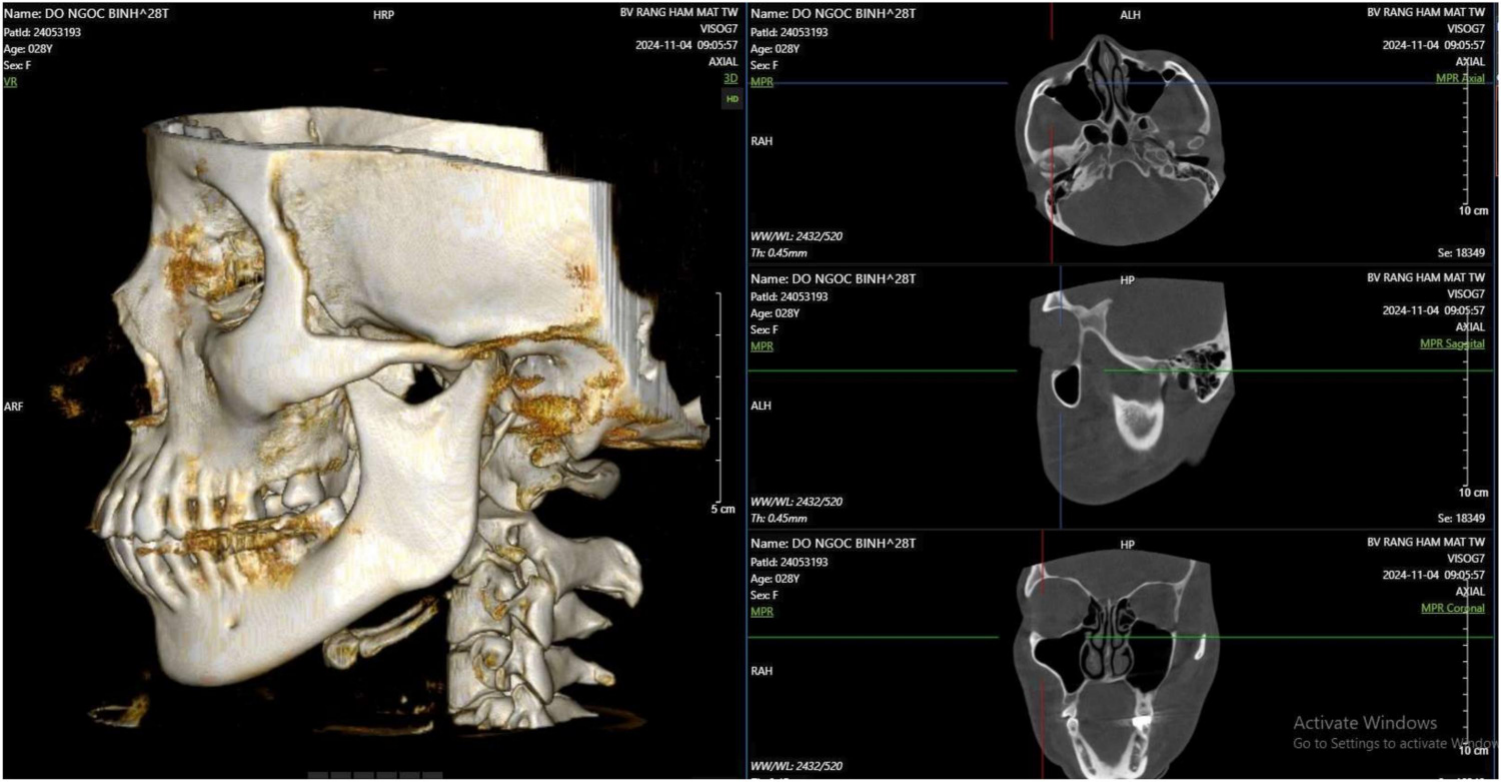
Reorientation of the CBCT axial plane to align with the Frankfort Horizontal (FH) plane.
